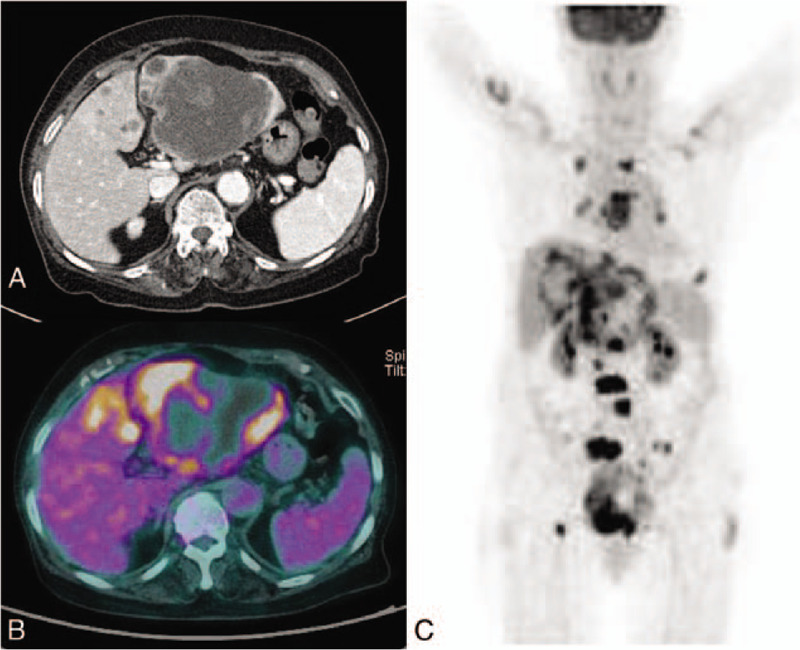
Abdominal computed tomography (CT) and Positron emission tomography-computed tomography (PET-CT). (A) About 10 cm sized, relatively well-defined hepatic mass with lobulated margin and peripheral rim enhancement. Multiple variable sized hepatic mass lesions showing similar characteristic were detected at left lobe. (B) and (C) ^18^F-fluorodeoxyglucose (FDG)-avid malignancy in left lobe of liver with multiple intrahepatic metastasis. Multiple lung, bone, right pleural metastasis, and peritoneal carcinomatosis were also shown.
